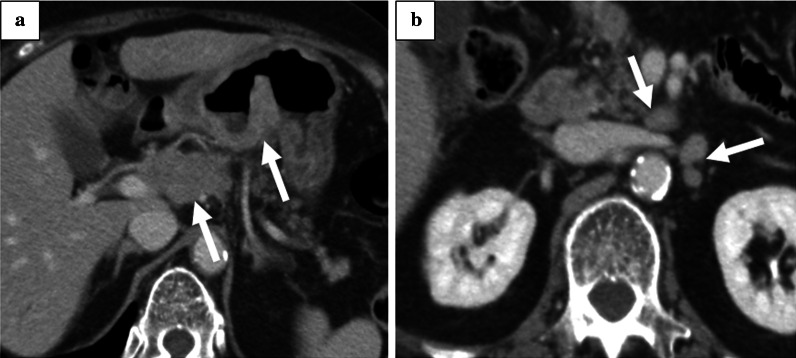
Contrast-enhanced CT at the time of diagnosis. a Thicker gastric wall and enlarged regional lymph nodes (No. 8a). b High-density and enlarged No. 16a2 lymph nodes
